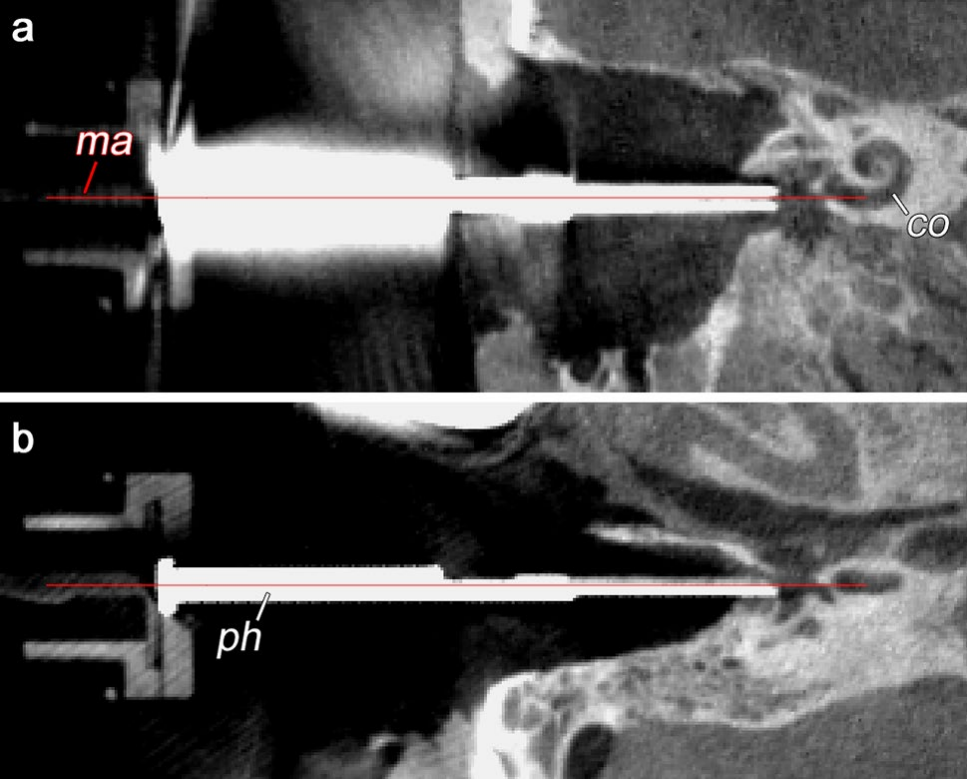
CBCT image of the CHD in its final position aiming toward the cochlea (co). Main axis (ma) of the probe holder (ph) is visualized (red line)
